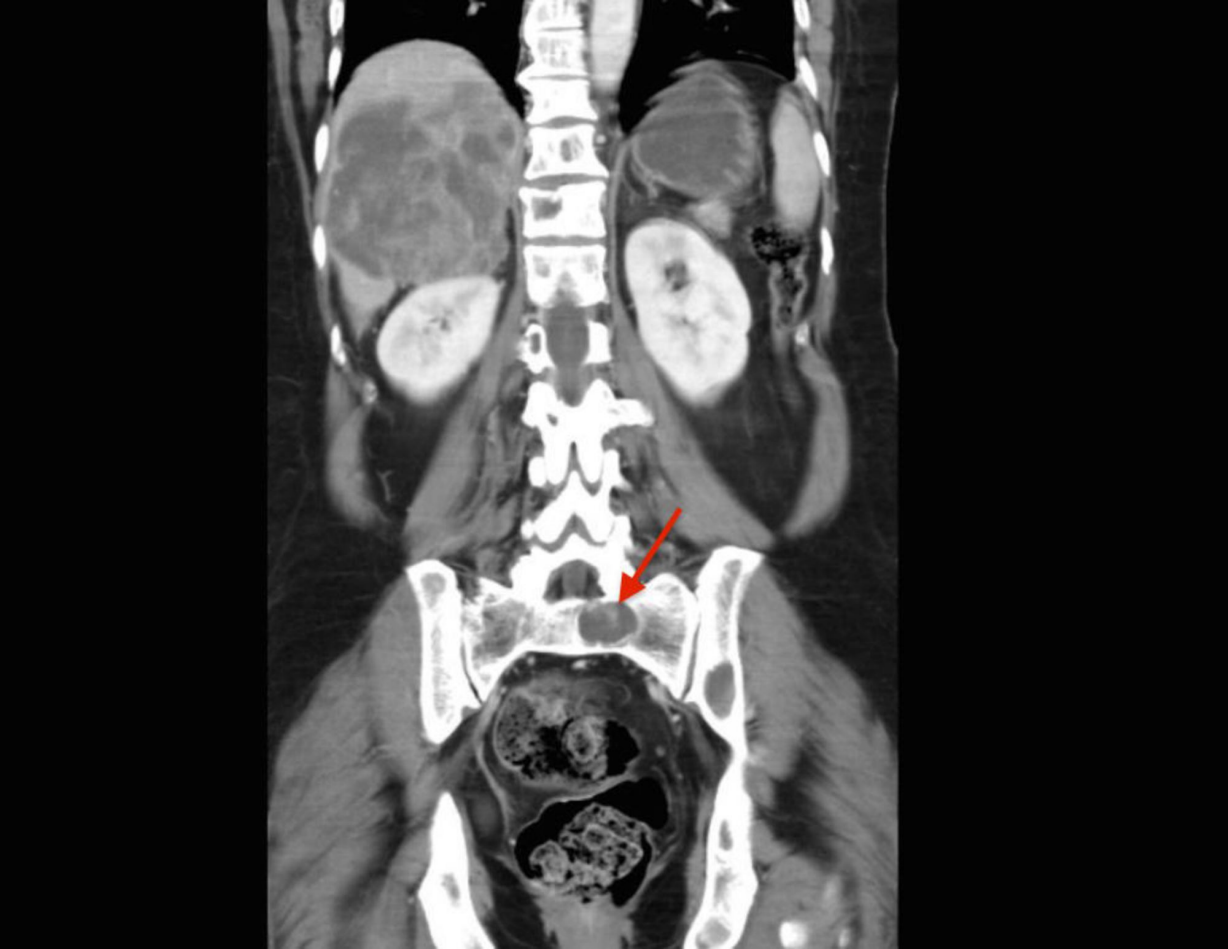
Coronal section of the patient's CT scan showing a lytic lesion in the left sacrum of the patient (red arrow) CT: computed tomography
